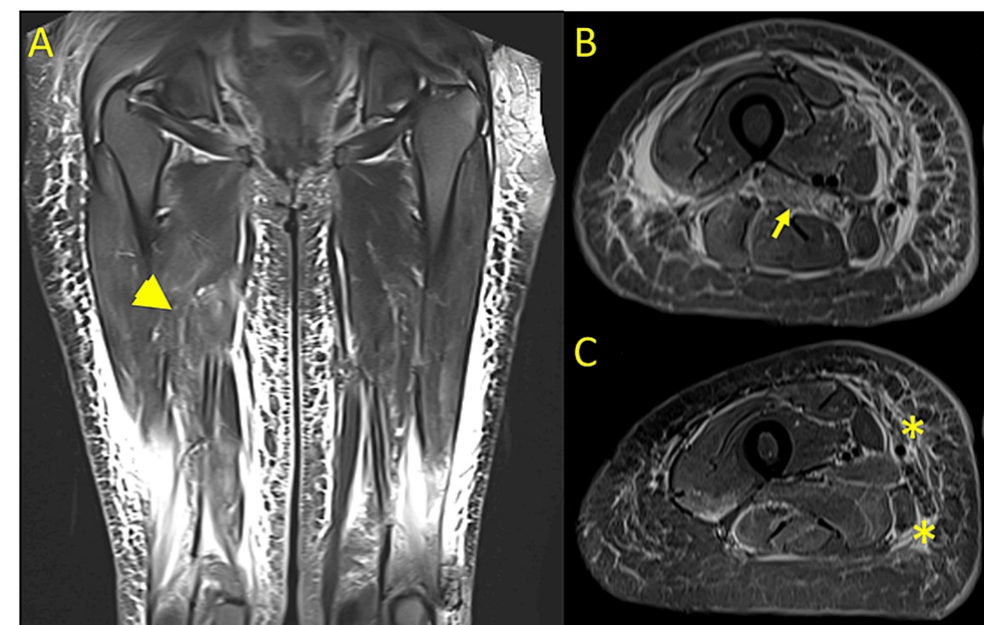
Lower limb magnetic resonance imaging. A. Contrast-enhanced T2 coronal view of both thighs. There's muscle edema predominantly in the right quadriceps (arrowhead). B. Contrast-enhanced T1 TIRM view of the left thigh showing muscle edema (arrow). C. Contrast-enhanced T2 view of the left thigh showing edema in the middle muscle compartment and subcutaneous tissue (asterisk). TIRM: turbo inversion recovery magnitude
